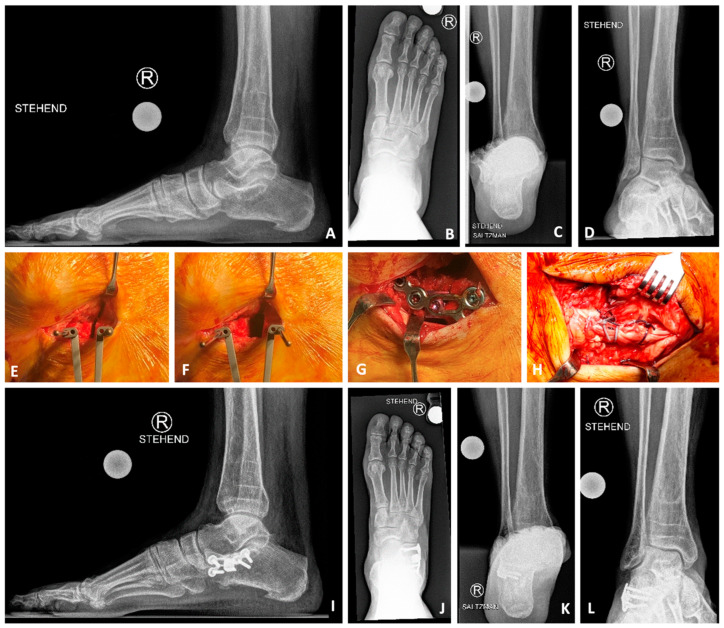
Lateral calcaneal lengthening osteotomy (LCLOT) with anatomical plate and titanium wedge. Sixty-four-year-old male with painful severe pes planovalgus and abductus right foot due to posterior tibial insufficiency with deltoid and spring ligament lesion. Treatment by LCLOT with anatomic Aptus-LCLOT-Plate (Medartis, Basel, Switzerland) and deltoid/spring ligament reconstruction as well as flexor digitorum longus (FDL) to posterior tibial (PT) tendon transfer. (A–D) preoperative X-rays: lateral foot, AP foot, Saltzman view, mortise view, (E–H): intraoperative pictures ((E) LCLOT with K-wire-forceps, (F) open of the LCLOT; (G) Aptus-LCLOT-Plate with titanium-wedge (Medartis, Basel, Switzerland) and spongiotic iliac-crest-autograft; (H) medial soft tissues reconstruction); (I–L) postoperative X-rays: 6 months postoperative with three-dimensional improvement of foot position and painfree patient.
